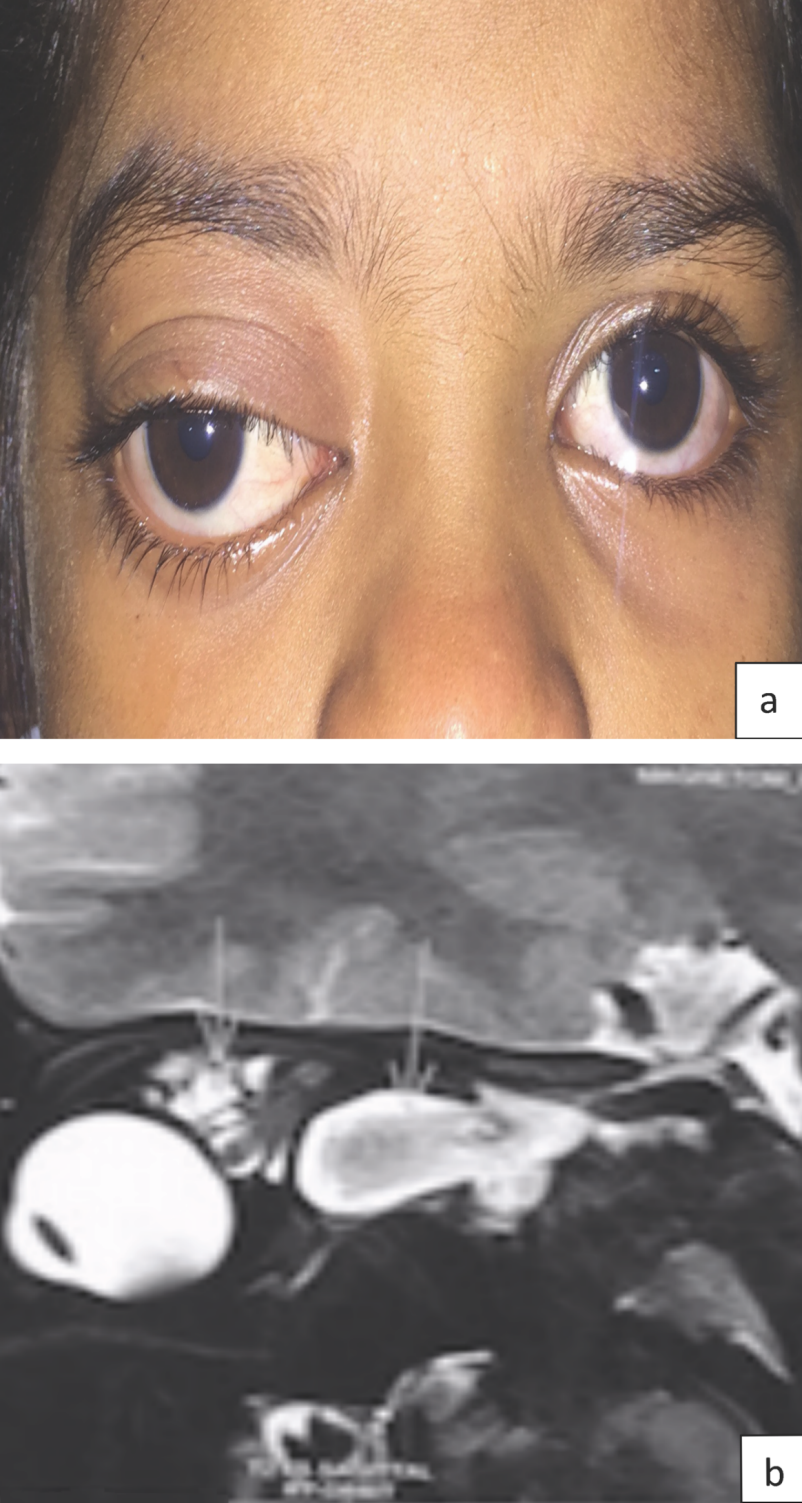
a) Clinical picture of the patient with down and out proptosis; b) MRI orbit sagittal view showing cystic component of lymphangioma above and below the optic nerve
